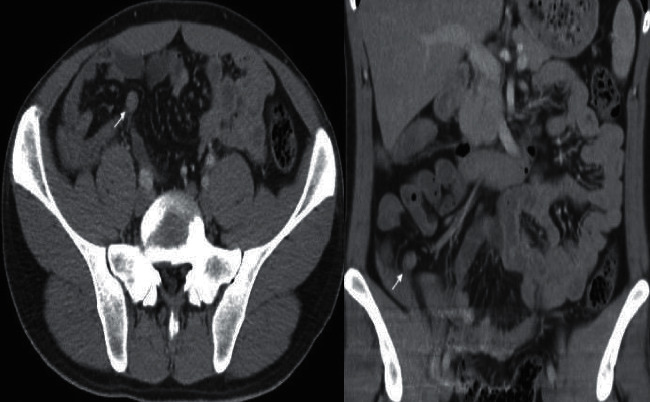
A suspicious mass is seen in the appendix in the axial and coronal CT scan of the same patient in the marked area.
